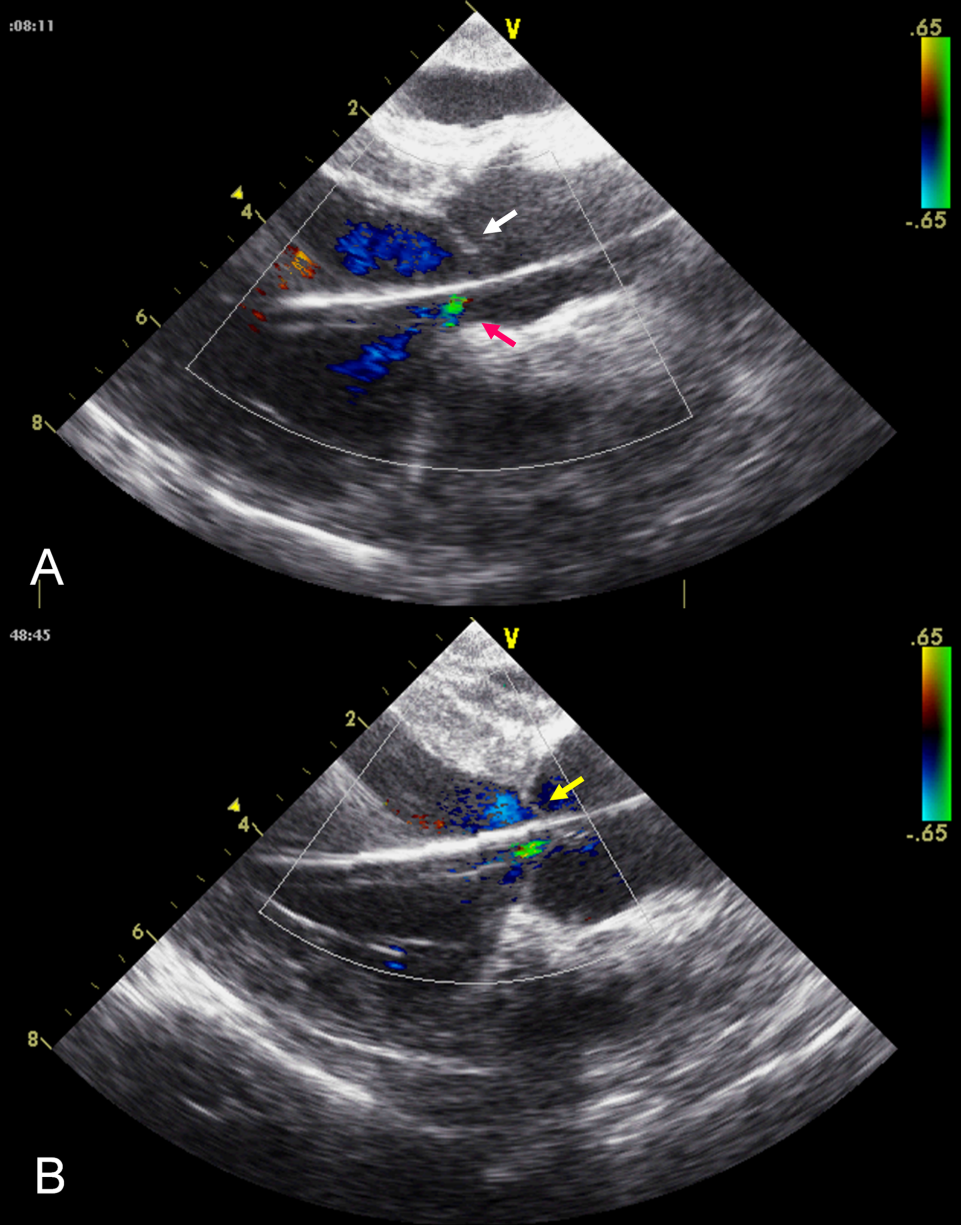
Echocardiograms after insertion of the drainage catheter. A. Echocardiogram showing an intact left coronary leaflet (white arrow) and right coronary leaflet compressed by the drainage catheter, generating moderate eccentric aortic insufficiency (red arrow). B. Echocardiogram showing that release of the vascular stent keeps the drainage catheter in the center of three valve leaflets. Trivial centric aortic insufficiency was observed in this case (yellow arrow).
